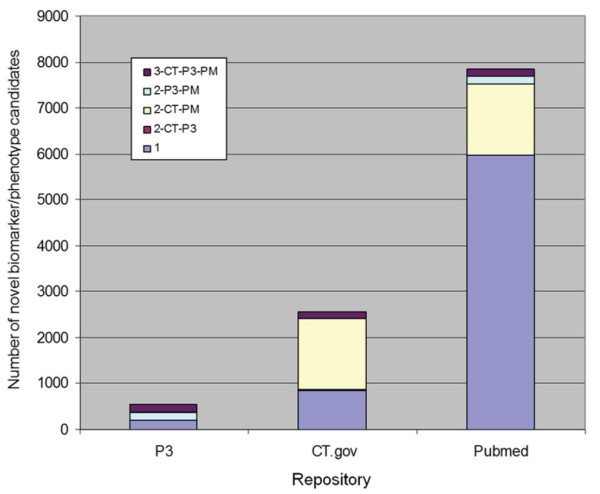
Novel candidates repository overlap. Overlap between novel InfoCodex biomarker/phenotype candidates from PubMed (PM), ClinicalTrials.gov (CT), and Merck internal research documents (P3). Lavender shading: found in one repository only; dark violet shading: found in all three; others: found in two.
